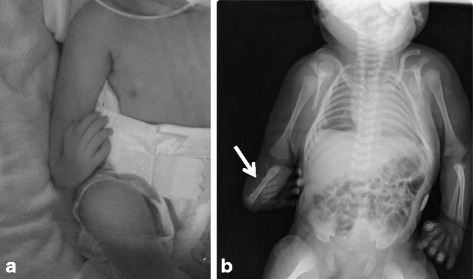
a The neonate’s physical examination reveal bend and contracture of right wrist joint. b A right radial ray defect is observed on this x-ray (*arrow*)
